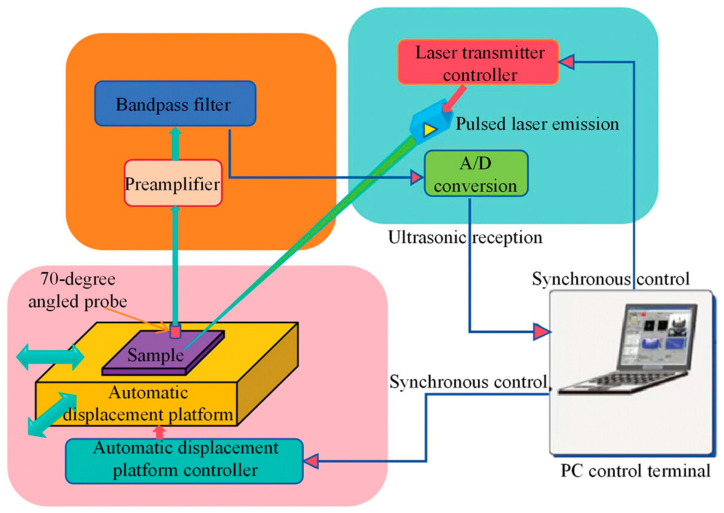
Laser ultrasonic testing system. Adapted from [149].
